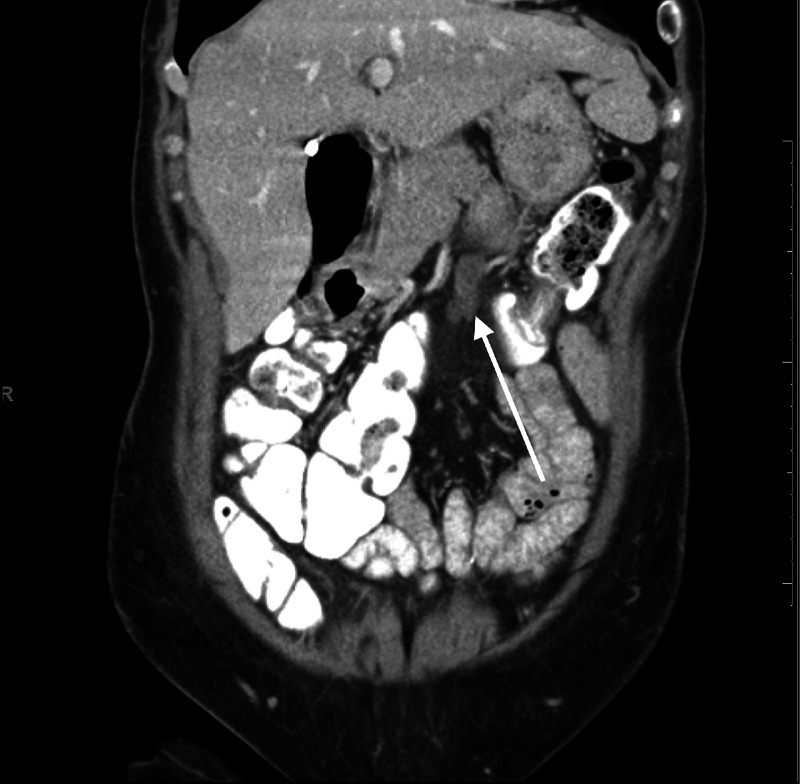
Diagnostic computed tomography Pre-intervention computed tomography of abdomen and pelvis with IV and oral contrast demonstrating a stable, previously reported 3.2 cm x 1.2 cm x 2.0 cm soft tissue mass of the anterior mesentery in the upper abdomen as demonstrated by arrow.
